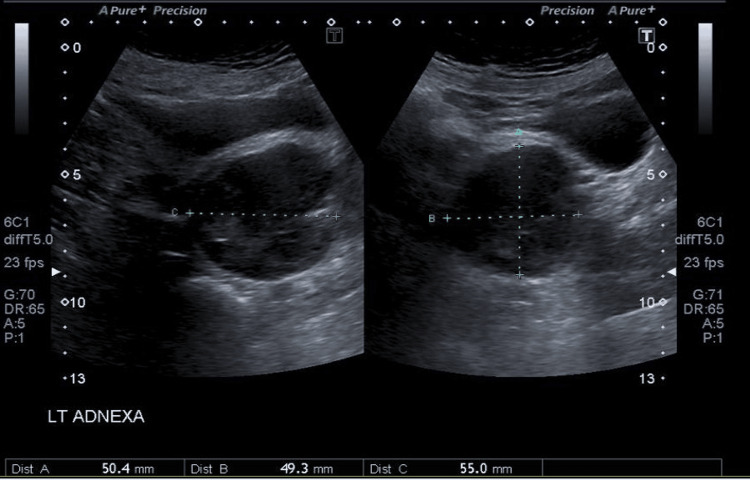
Ultrasound image demonstrating 50x49x55 mm cystic mass adjacent to left ovary. Hyper echoic areas in the cyst are consistent with features of dermoid cyst.
